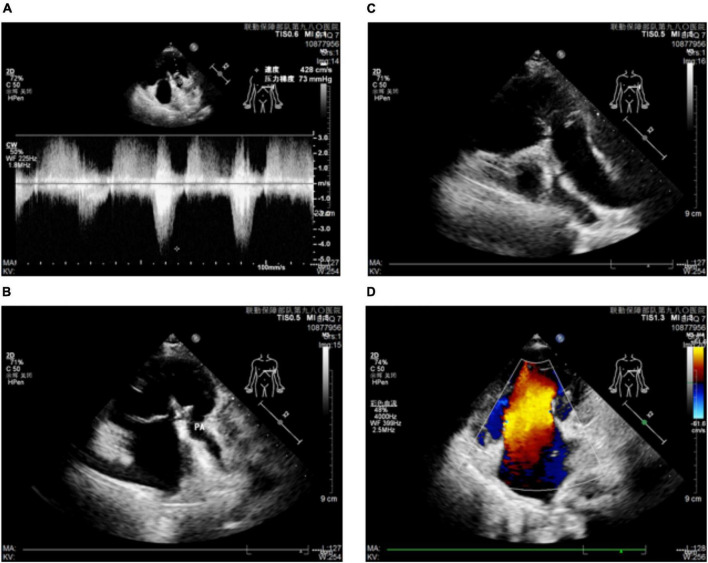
Ultrasound imaging of the patient’s heart. (A,B) Patient’s atrial ventricle and pulmonary artery imaging and pressure. (C) Images of the patient’s atrium ventricle and aorta. (D) Atrial ventricular flow imaging of the patient.
